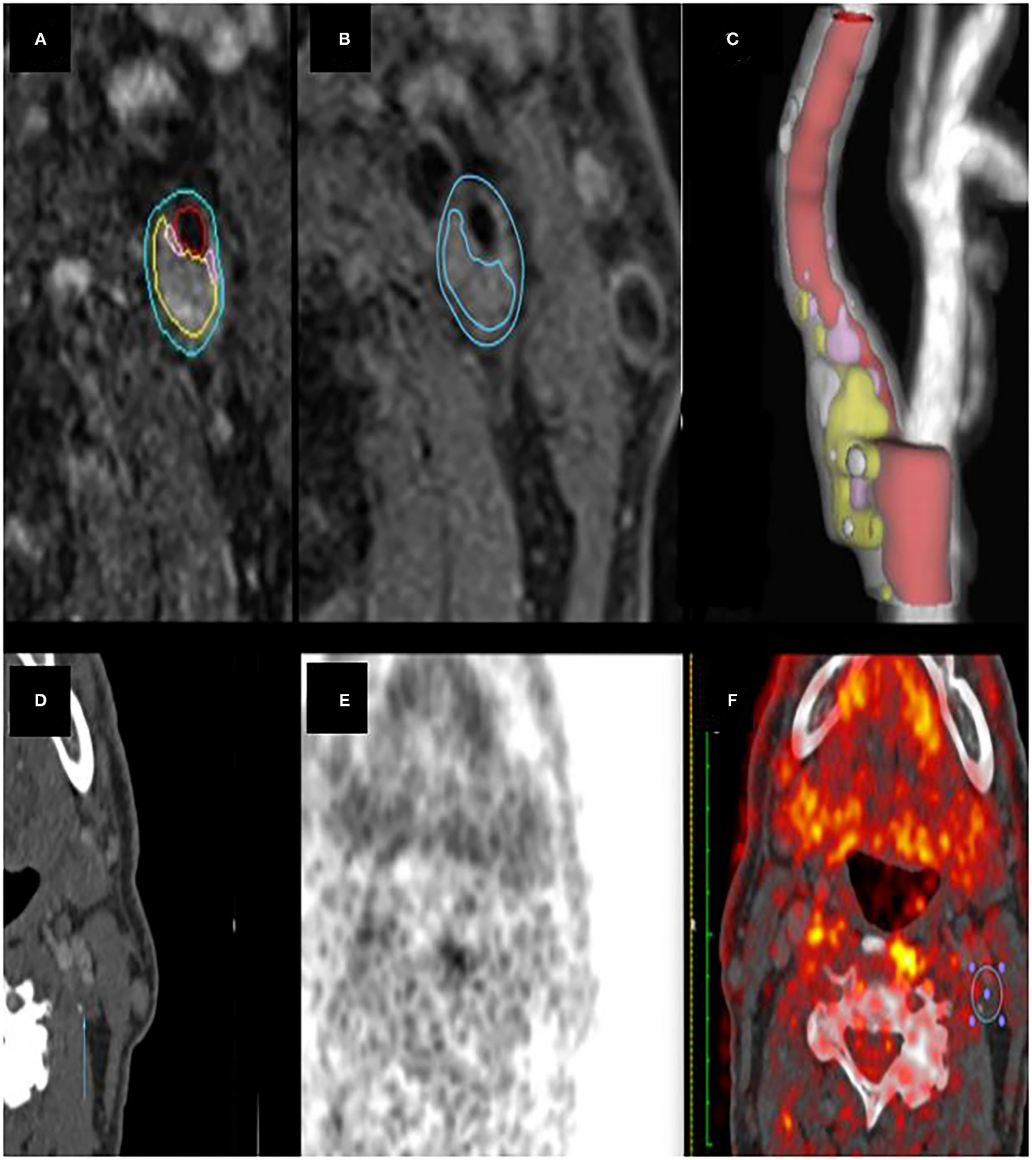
(A) carotid MRI semi-automatic segmentation of lumen and vessel wall. (B) semi-automatic plaque characterisation of an LRNC area. (C) ICA plaque 3D Volume Rendering. (D–F) CT and PET images of the same plaque area. The ROI (F) shows where SUV_max_ was measured.
